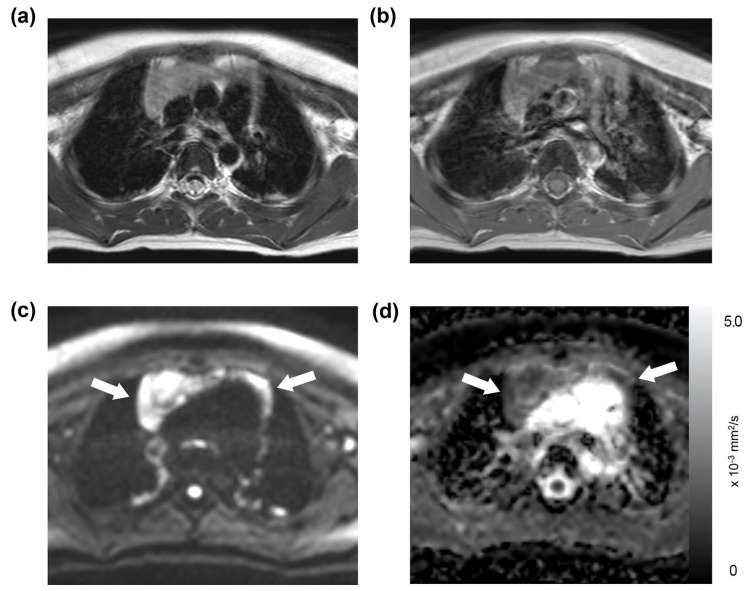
Diffuse restricted diffusion in the thymus: a 4-year-old boy with a history of mediastinal neuroblastoma. This patient underwent an MRI on a Siemens A scanner, with their laboratory data showing a WBC count of 8.43 × 10^3^/μL, an absolute neutrophil count of 4.37 × 10^3^/μL, an absolute lymphocyte count of 3.15 × 10^3^/μL, and a platelet count of 215 × 10^3^/μL. The thymus was found to be slightly hyperintense on the T2-weighted image (a) and isointense on the T1-weighted image (b). Diffuse restricted diffusion was present on the DWI (c, arrows), which corresponds to low-signal intensity in the ADC map with a mean ADC value of 1.23 × 10^−3^ mm^2^/s (d, arrows).
